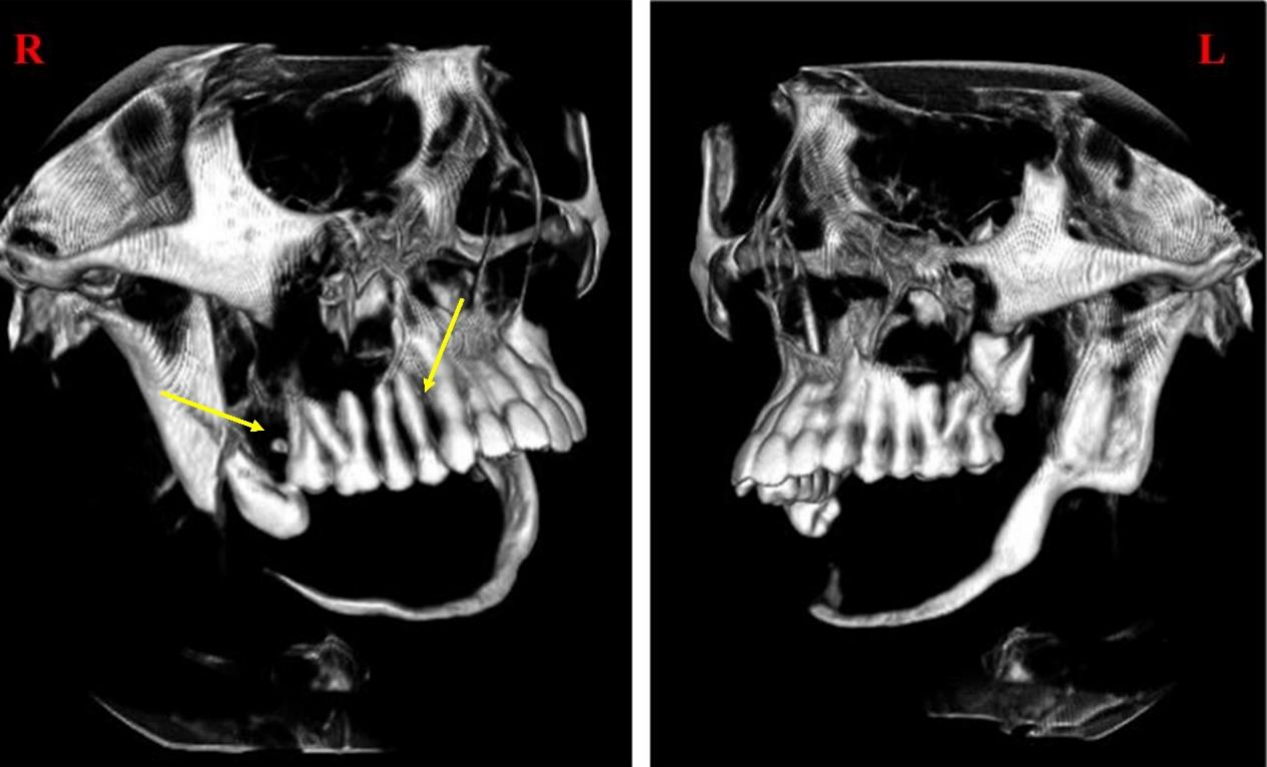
Sagittal 3D-CBCT image showing hypodense areas in right posterior maxillary region (yellow arrows) and completely resorbed mandible.
